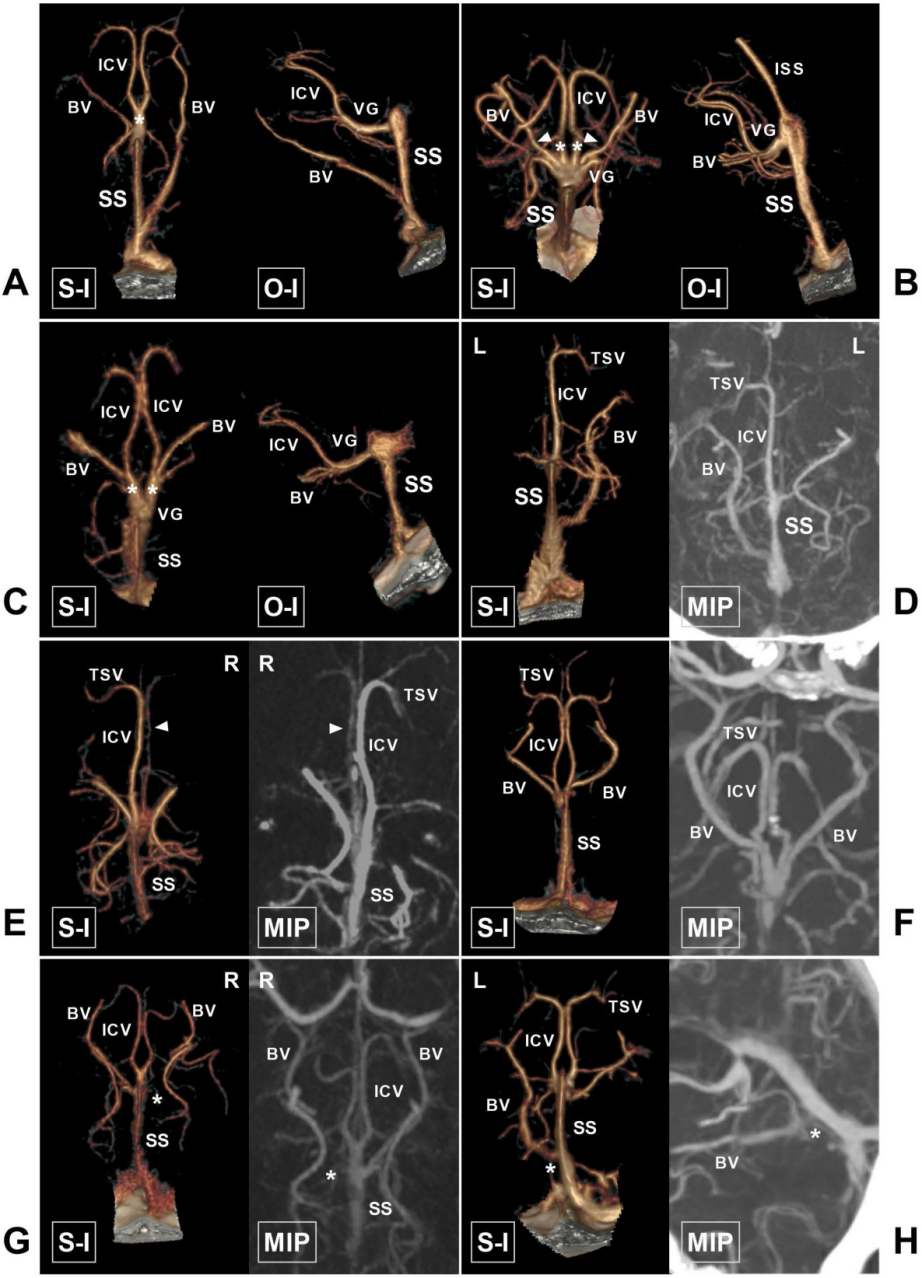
The ICV and BV and their draining characteristics into the VG and SS. A, CTA shows that the bilateral ICVs merge into one trunk (asterisk) before draining into the VG. B, CTA shows that the bilateral ICVs (asterisk) and BVs (arrowhead) drain into the VG. C, CTA shows that the ipsilateral ICV and BV merge into one trunk (asterisk) before draining into the VG. D, CTA and MIP show that the left ICV is absent. E, CTA and MIP show that the right ICV (arrowhead) is dysplastic. F, CTA and MIP show that the bilateral BVs are well developed. G, CTA and MIP show that the right BV is dysplastic (asterisk). H, CTA and MIP show that the left BV drains directly into the SS (asterisk). Abbreviations: BV, basal vein; CTA, computed tomography angiography; ICV, internal cerebral vein; ISS, inferior sagittal sinus; MIP, maximum intensity projection; O-I, outside to inside; S-I, superior to inferior; SS, straight sinus; TSV, thalamostriate vein; L, left; R, right; VG, vein of Galen.
